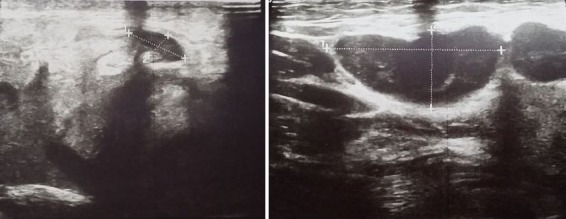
Cervical ultrasound showing cervical lymphadenopathy of hypoechogenic ovoid echostructures
